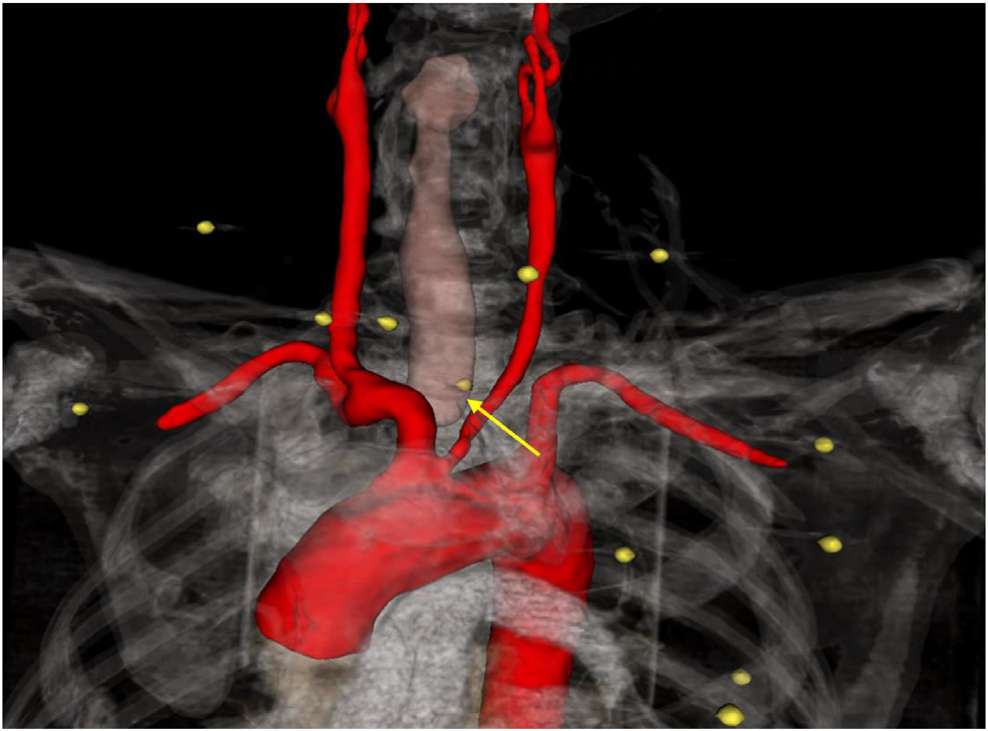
CTA image of the neck demonstrating a metallic pellet adjacent to the trachea (yellow arrow).•Post‑tracheal, anterior to the esophagus, without direct perforation.•Associated finding: superior pneumomediastinum, suggesting a possible high aerodigestive tract breach.
